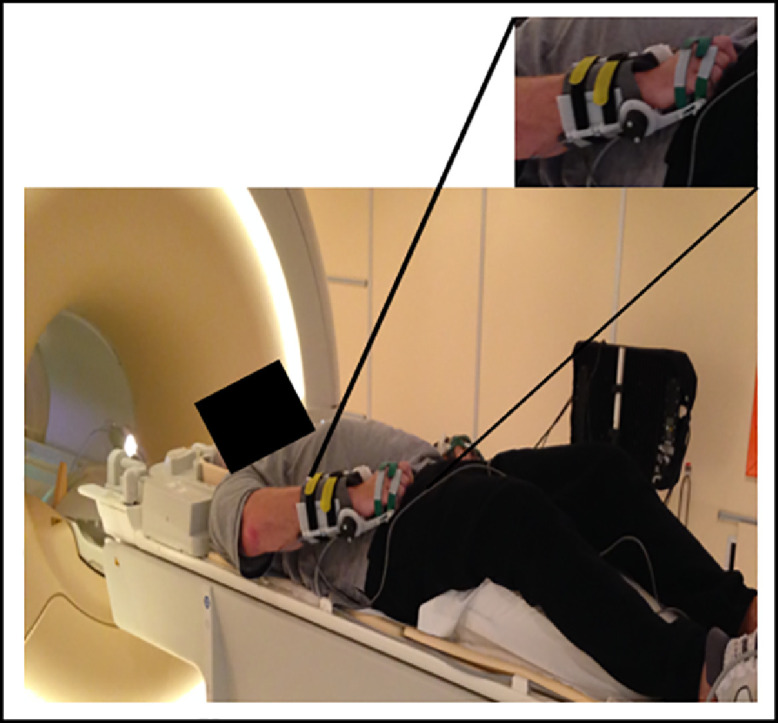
Picture of experimental setup showing homemade MR-compatible goniometer attached to patient's wrist in MRI scanner room. Patient's wrist is at rest, corresponding to an angle of 0°.
